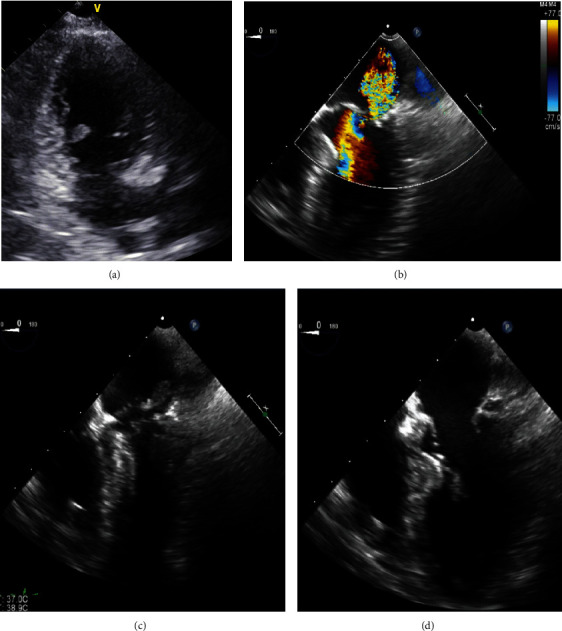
Transthoracic and transesophageal echocardiogram on day 5. (a) A fluttering mass in the left ventricle (LV), suggestive of papillary muscle rupture (white arrow) on apical two-chamber view. (b) Systolic-phase view with color doppler showing systolic jet towards the probe, indicative of mitral regurgitation. (c, d) Systolic- and diastolic-phase images of the completely ruptured posterior papillary muscle moving between the LV and left atrium (white arrow).
